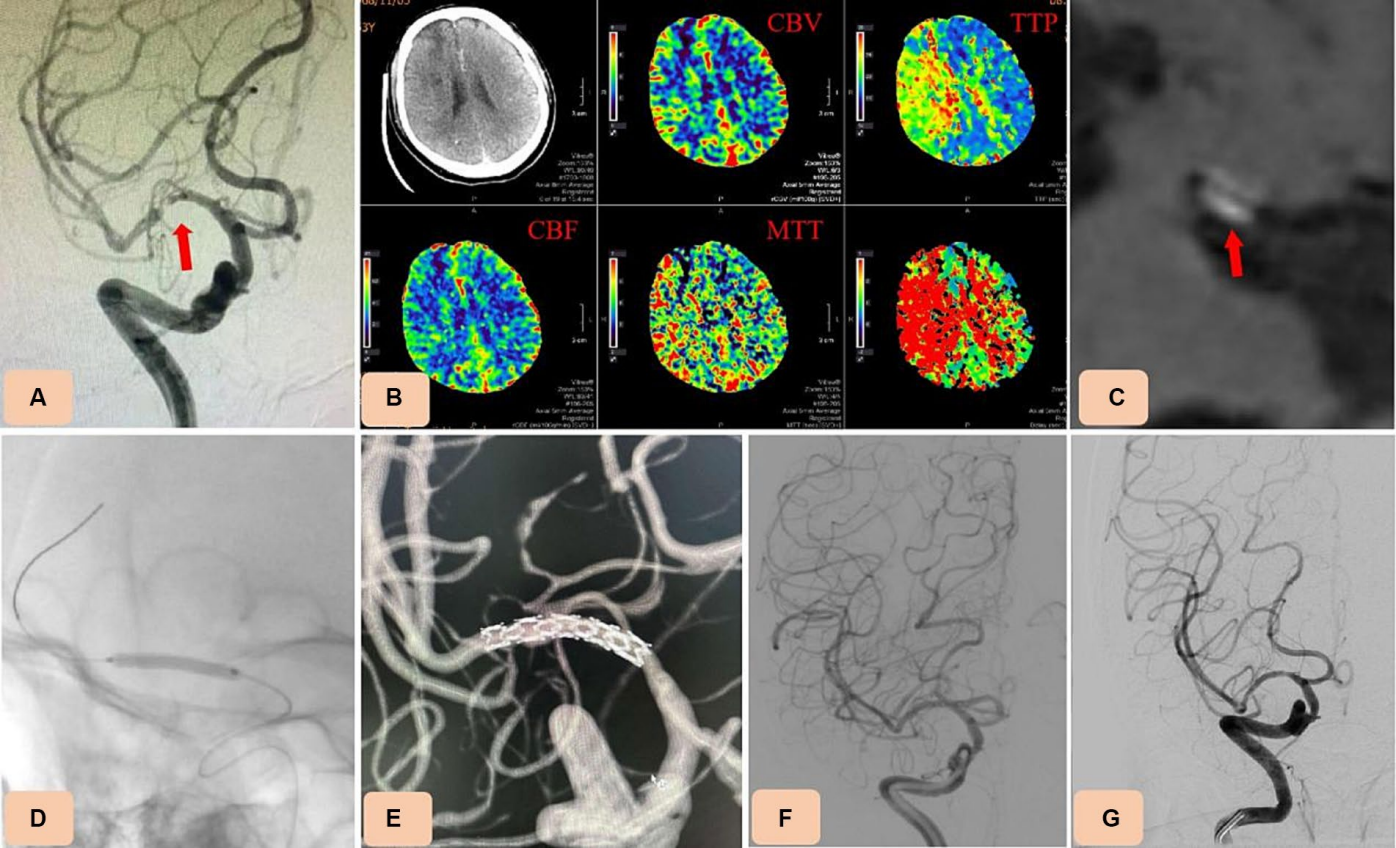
Case 3. Percutaneous transluminal angioplasty and stenting (PTAS) for severe M1 segment stenosis of the right middle cerebral artery (MCA) with the novel drug-eluting stent (DES), NOVA. (A) Preprocedural digital subtraction angiography showing severe M1 segment stenosis of the right MCA (arrow). (B) Computed tomography perfusion (CTP) showing hypoperfusion on the right MCA territory (increase of Time-to-peak (TTP) and Mean transit time (MTT)). (C) High resolution magnetic resonance imaging (HR-MRI) revealing unstable plaques (arrow). (D,E) A selected NOVA stent (2.5 mm × 12 mm) was advanced across the stenotic vessel and inserted by inflating the balloon. (F) Postoperative angiography confirmed the in-stent patency, with no residual stenosis. (G) Thirteen-month follow-up angiography showing no in-stent restenosis.
